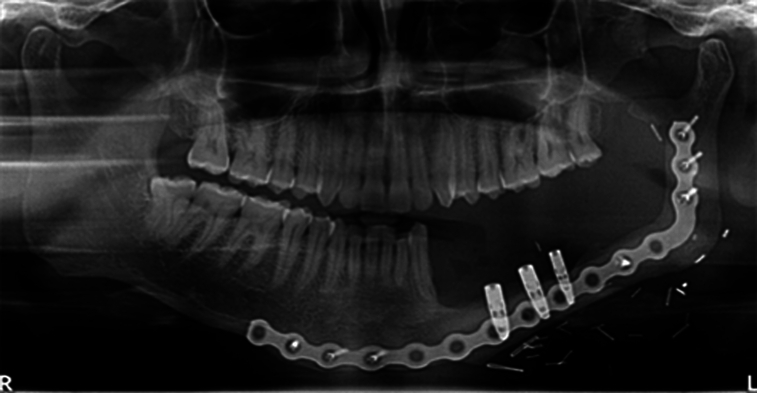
Panoramic radiograph of Implant placement in reconstructed fibula.
